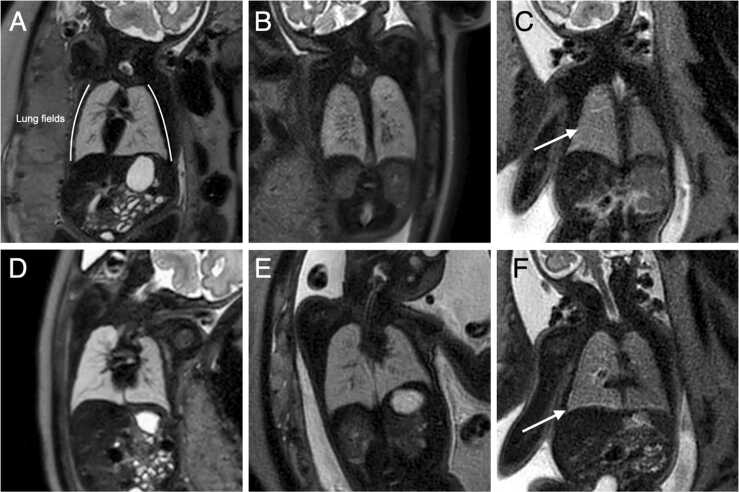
Example T2-weighted fetal MRI images. Panels A and D show images from two cases reported as showing no evidence of PL; panels B and E show images from two cases showing increased generalised lung heterogeneity suspicious of PL; panels C and F show images from a single cases with diagnostic features of PL, including linear hyperintense signal extending from the hilums (C, arrowed) and pleural effusions (F, arrowed). *MRI* magnetic resonance, *PL* pulmonary lymphangiectasis
